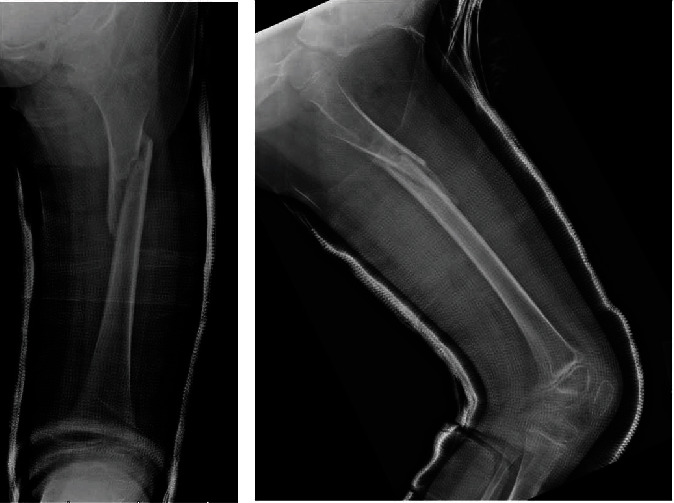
Healing X-rays from clinic 5 weeks after spica cast application.
